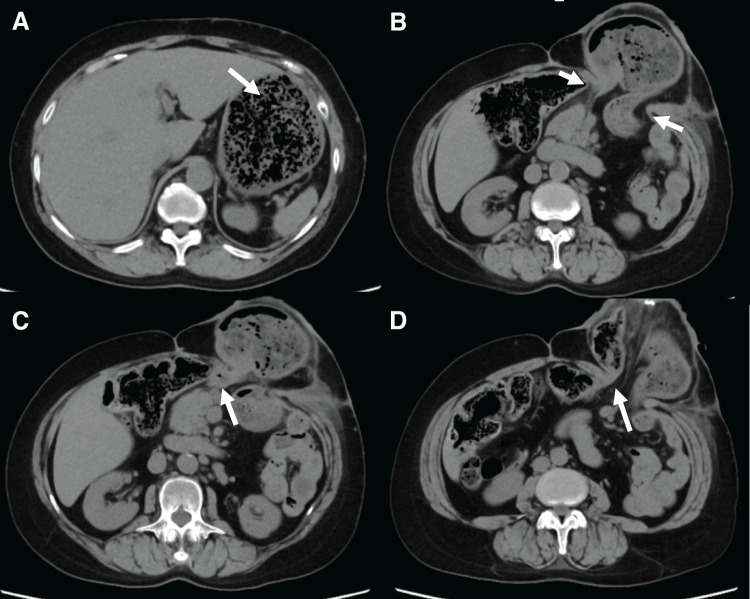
Preoperative CT findings showing gastric incarceration in a parastomal hernia. Axial CT images (A–D) are shown from cranial to caudal levels. (A) Marked gastric dilatation (arrow) was observed. (B) The gastric body was incarcerated and herniating through the parastomal defect (arrows). (C) Thickening of the gastric wall was evident in the antrum (arrow). (D) The lifted colon (arrow) was identified at the caudomedial side of the hernia orifice.
